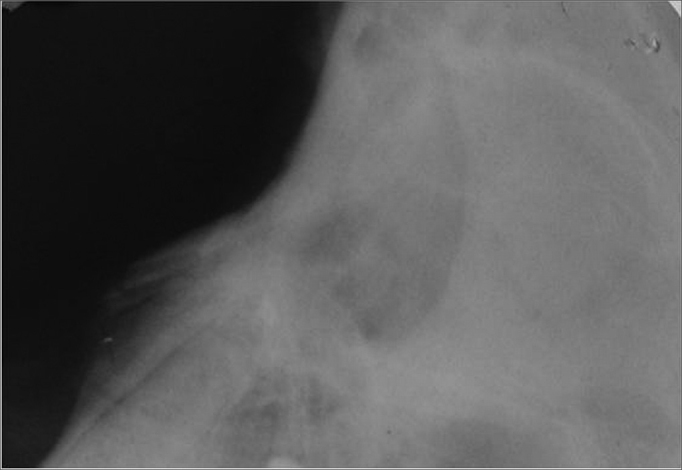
Lateral nose radiograph after 270 days.
